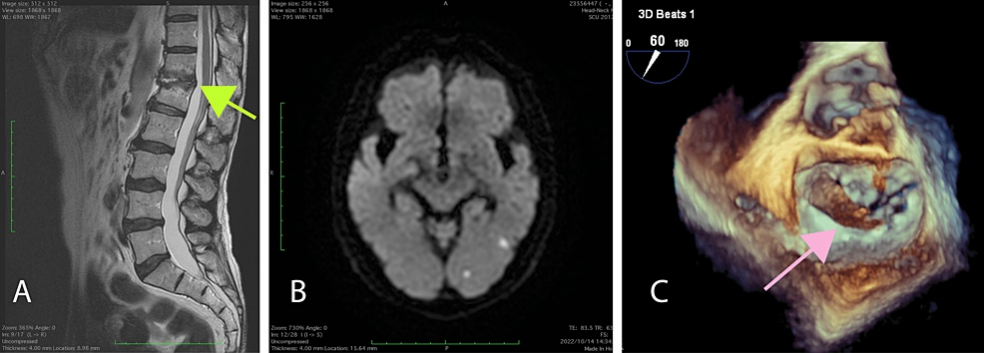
Preoperative findings (A) Preoperative lumbar MRI. Destruction of the first lumbar vertebrae was observed, and pyogenic spondylitis was suspected. The green arrow shows the fractured site. (B) Brain MRI. Diffusion MRI showed multiple infarction sites. (C) Transesophageal echocardiogram. 30-mm-sized vegetation was detected at the mitral valve anterior commissure. The pink arrow shows the vegetation MRI: magnetic resonance imaging
